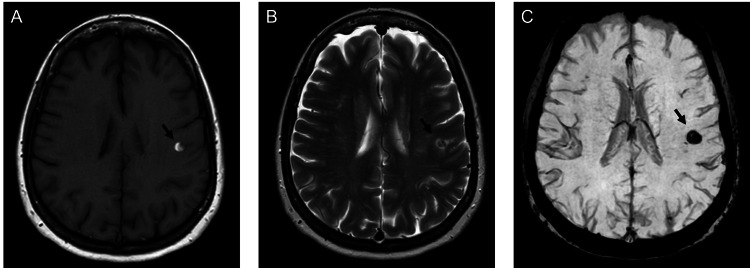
MRI of the brain obtained on 3 Tesla scanner (A) Axial T1 showing subacute hemorrhage in the left frontotemporal lobe; (B) Axial T2 showing heterogenous “popcorn” lesion with perifocal hemorrhage in the left frontotemporal lobe; (C) Axial susceptible weighted imaging showing blooming artifact in the left frontotemporal lobe. This is consistent with early subacute hemorrhage. Arrows are used where appropriate to indicate pathology.
